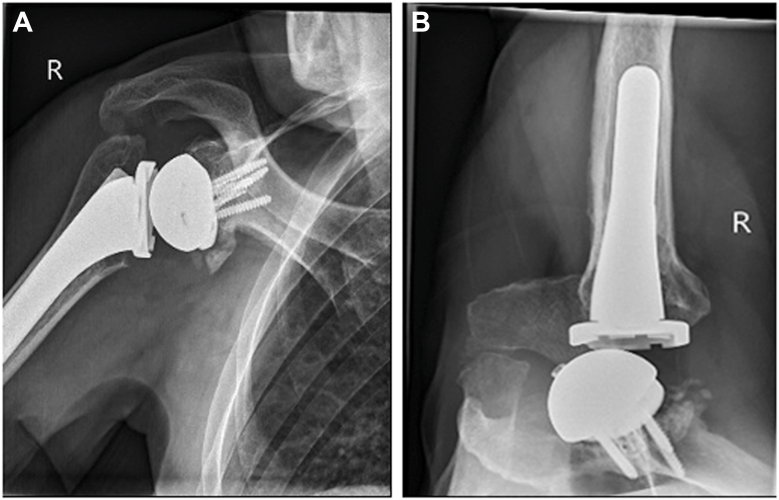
(A) 1-year postoperative radiographs, AP. (B) 1-year postoperative radiographs, axial. *AP*, anteroposterior.
